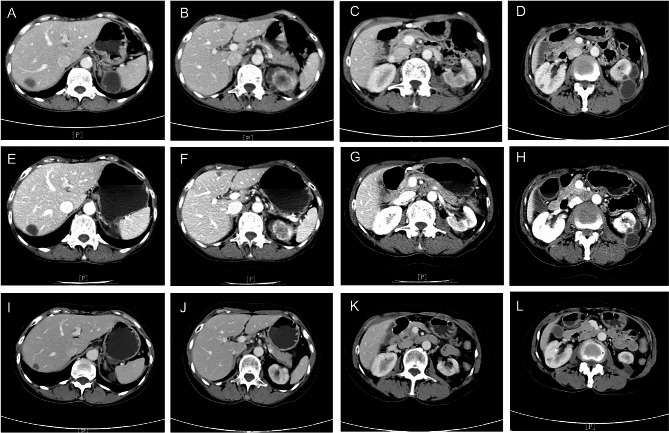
Serial contrast-enhanced abdominal CT scan after discontinuation of crizotinib. Panels A-D: One month after stopping crizotinib treatment, showing significant reduction of the size of cystic lesions. Panels E-H: Two months after stopping crizotinib treatment, showing continuous reduction of the cystic lesions. Panels I-L: Six months after stopping crizotinib treatment, demonstrating size of renal cysts significantly decreased and hepatic cyst nearly disappeared
